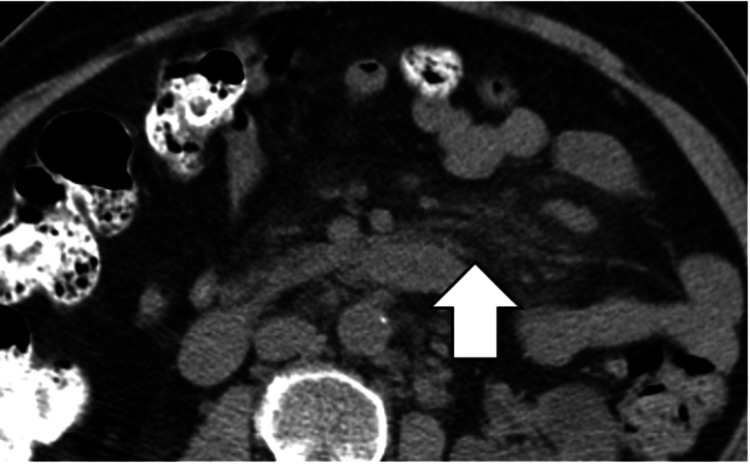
Mesenteric panniculitis. The CT image shows a misty appearance of the mesentery (white arrow) adjacent to the jejunum with small and prominent lymph nodes and a mild hyperattenuating pseudocapsule.
